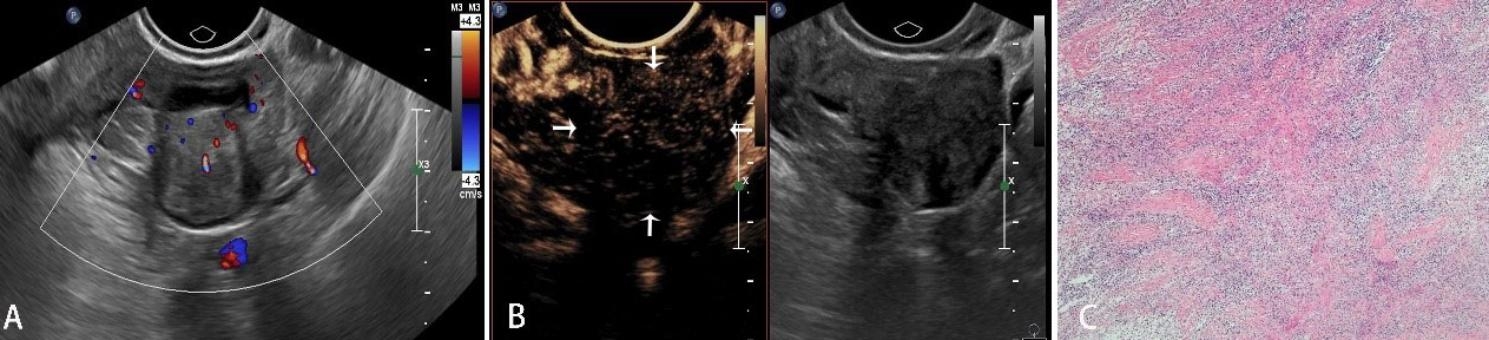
Transvaginal ultrasonogram and pathological image of a left adnexal mass in a 59-year-old patient. A: Conventional ultrasound showed that the mass had a maximum diameter of 2.7 cm, regular in shape. CDFI showed the short strip-shaped blood flow signal inside the mass, which was classified as 4 by O-RADS. B: CEUS showed hypoenhancement at 38s(arrow); it was downgraded to 3 by O-RADS combined with CEUS. C: The pathological results revealed that it was the left ovarian fibrotheca cell tumor (HE × 40)
